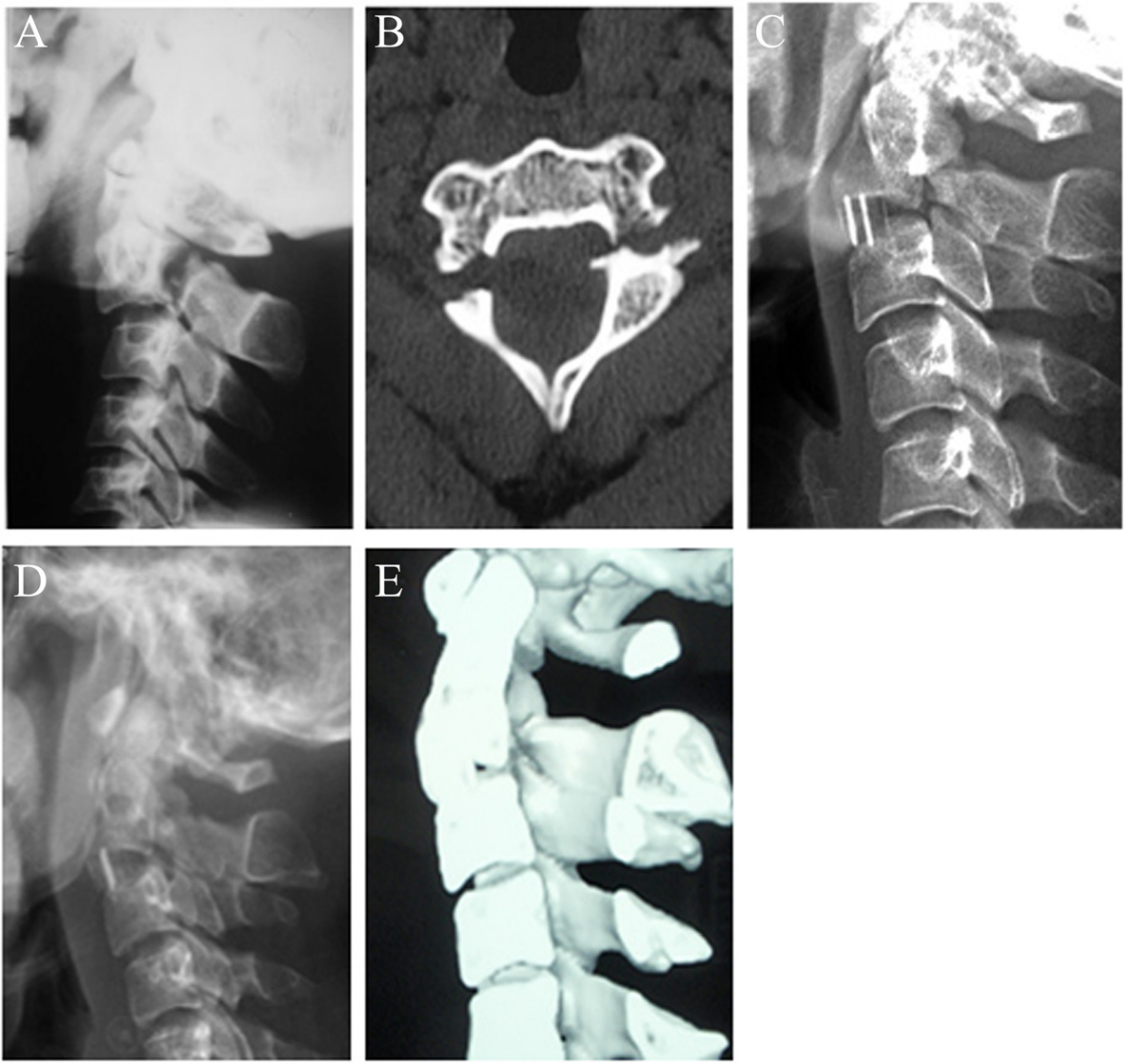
Images of a 26-year-old female patient. a and b, preoperative lateral X-ray and CT scans showing a type II hangman’s fracture with severe translation. c, Postoperative lateral X-ray showing minor implant migration at 1 week postoperatively. d, The X-ray showed the implant remained the same position during follow-up. e, Bone fusion was confirmed using CT scan at 4 months postoperatively
